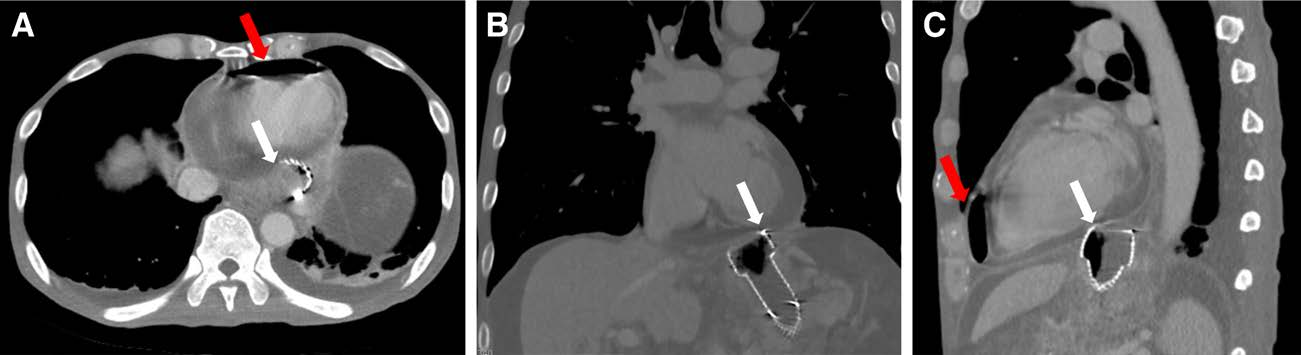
Chest CT images obtained at the initial presentation. (A) Axial view, (B) coronal view, and (C) sagittal view. Intrapericardial air is indicated by red arrows and white arrows indicate the esophageal stent closely abutting the pericardial contour. CT = computed tomography.
